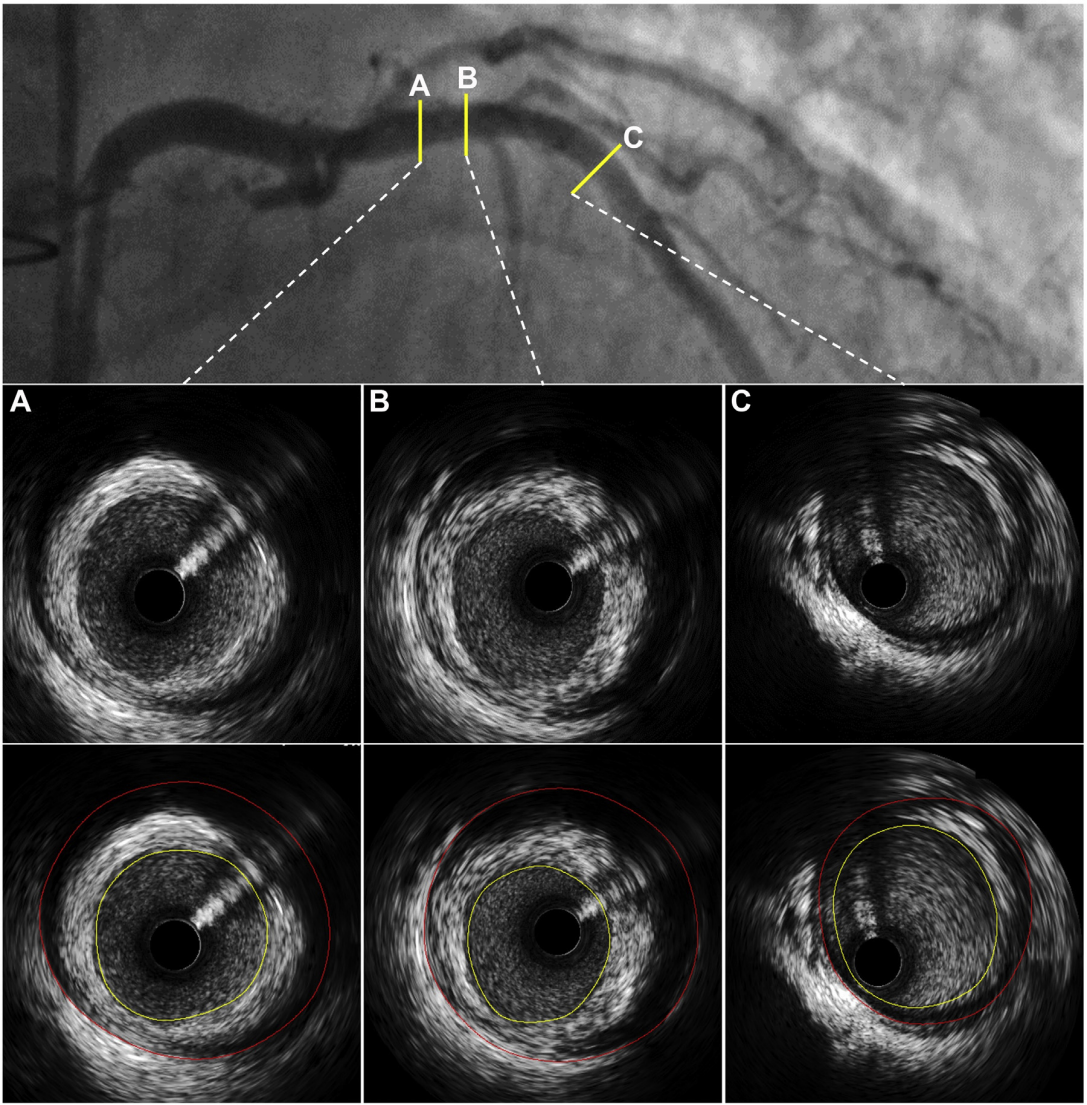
Discordance of coronary angiography and IVUS. The LAD appears to have minimal disease on angiography. IVUS reveals moderate concentric plaque (maximum plaque burden: 71%) in the proximal segment (panels A and B), as well as mild plaque in the mid segment (panel C). IVUS, intravascular ultrasound; LAD, left anterior descending.
